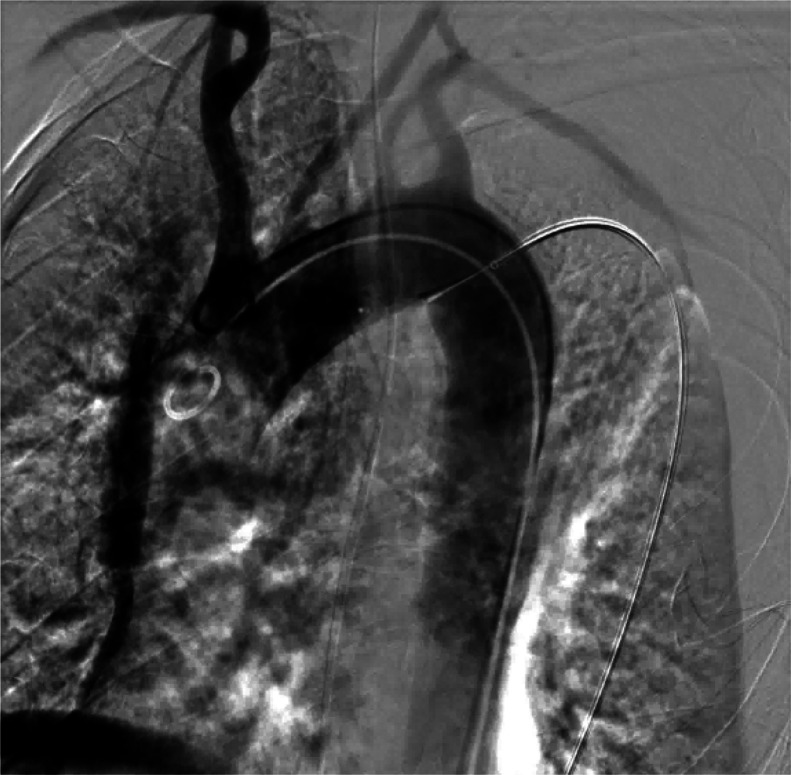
Postoperative chest CT re-examination; the arrow indicates the ventricular septal defect occluder.
